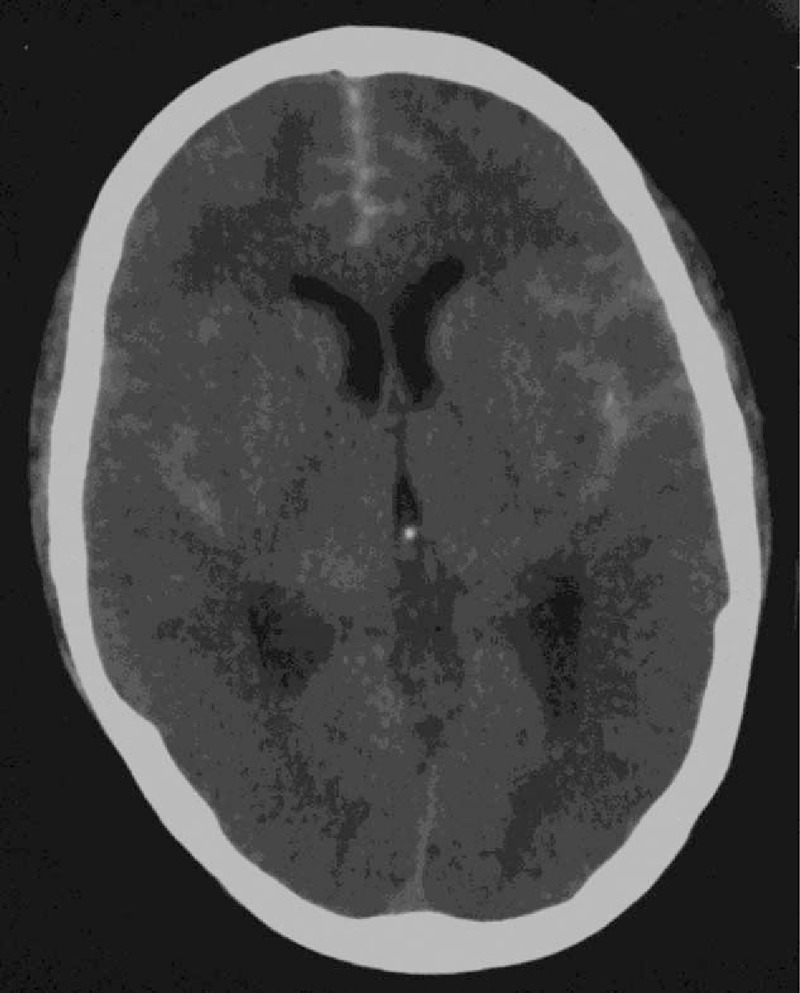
CT demonstrating subarachnoid hemorrhage. CT = computed tomography.
